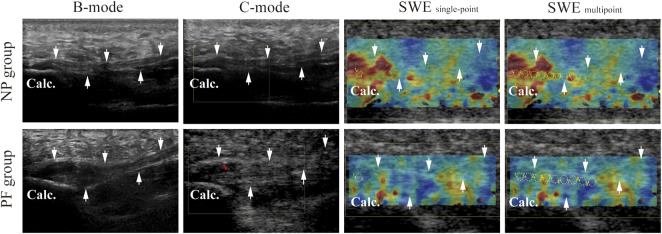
Acquisition plantar fascia images (arrows) of different ultrasound modalities in both NP group and PF group. Ultrasound modalities include B-mode, C-mode, and SWE. B-mode, and B-mode ultrasonography; C-mode, Color Doppler mode ultrasonography; SWE, shear wave elastography; NP group, non-pain group; PF group, plantar fasciopathy group; Calc, calcaneal.
